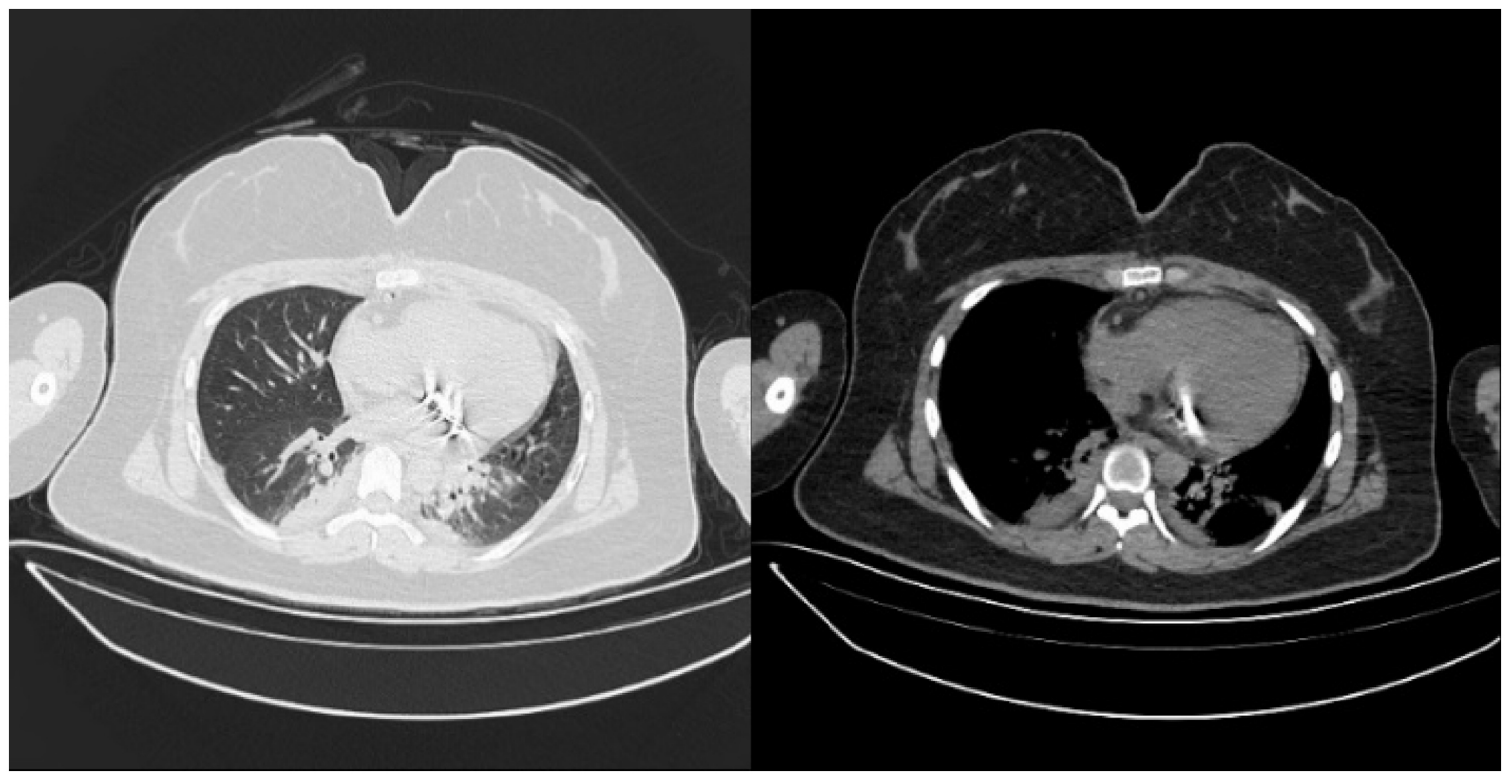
Postoperative chest computed tomography results. Complete resection of intracardiac tumor in the left ventricle, with involvement of the mitral valve. The mitral valve was removed during the surgery and replaced with a mechanical valve.
